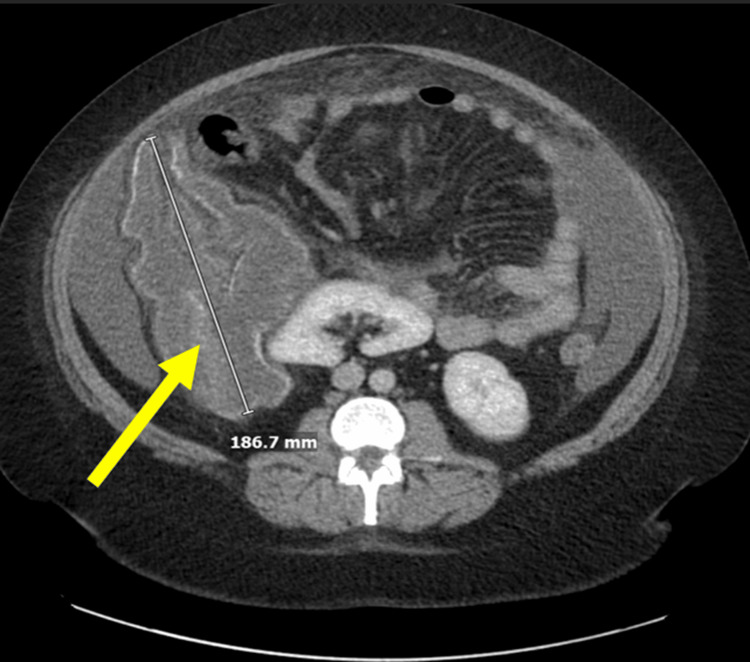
CT scan of the abdomen and pelvis showing a peripherally calcified, lobulated fluid collection (18.7 cm) inferior to the liver CT: computed tomography
